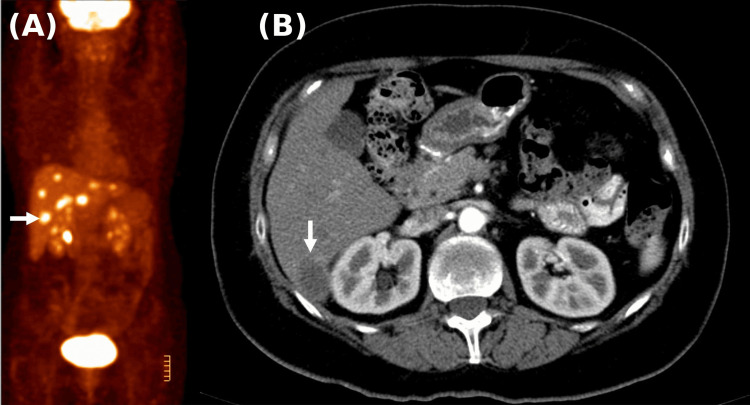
FDG-PET/CT imaging from 2010 demonstrating extensive bilobar hepatic metastases (A) Coronal FDG-PET image demonstrating multiple hypermetabolic foci throughout the liver (arrow), consistent with extensive bilobar metastatic disease. (B) Axial CT image showing a corresponding hypodense hepatic lesion in the right lobe of the liver (arrow), confirming metastatic involvement. FDG-PET/CT: fluorodeoxyglucose-positron emission tomography/computed tomography
